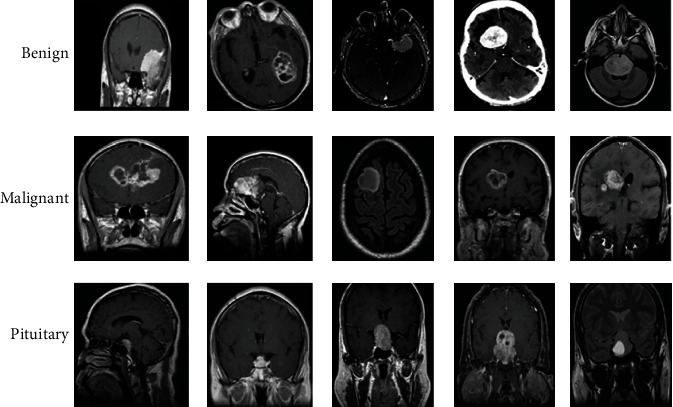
BraTS dataset sample brain MR images.
